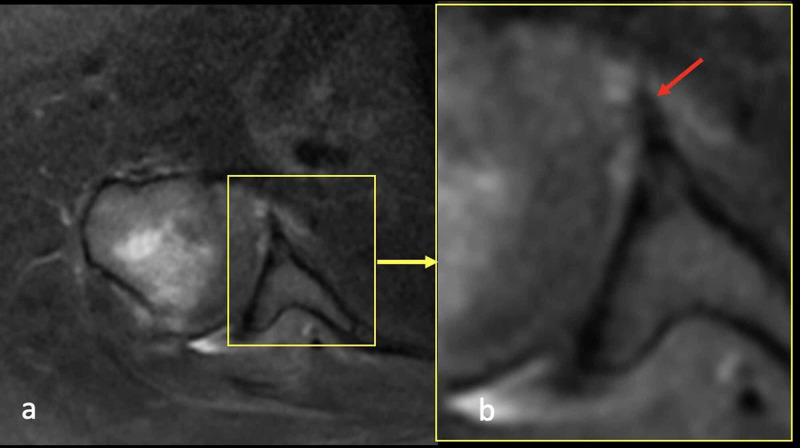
MRI images of case 1 (a) An axial image showing totally fixed LHBT to the labrum; (b) a magnification view of MRI showing the LHBT in the glenoid (red arrow). LHBT: long head of the biceps tendon.
